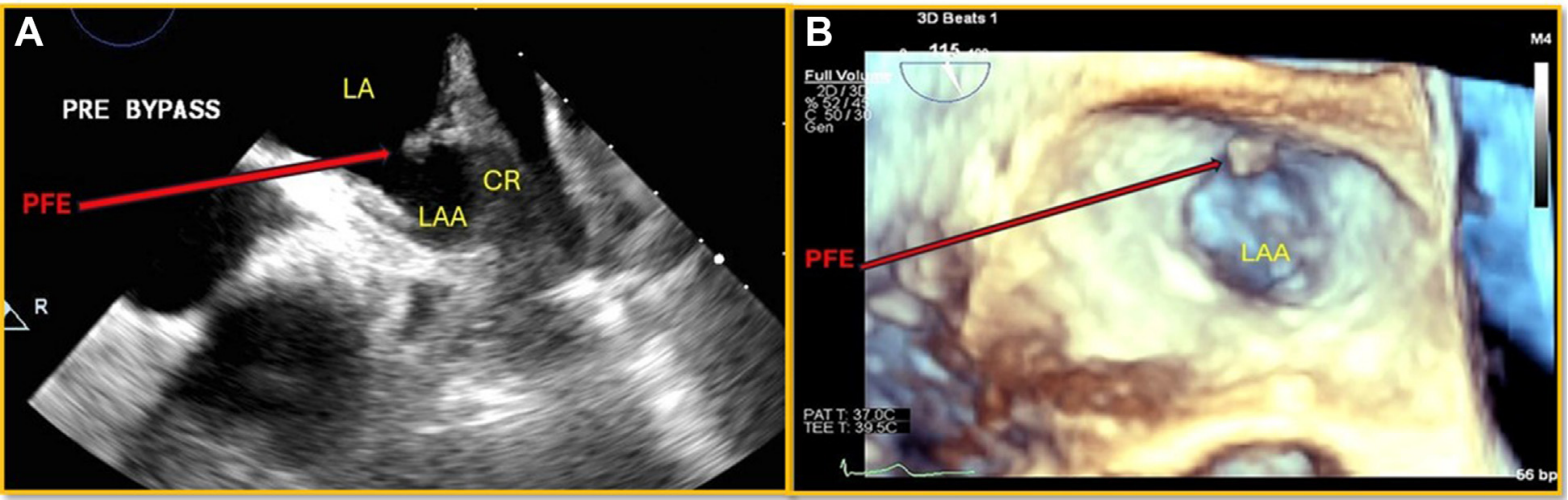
Transesophageal Echo (A) Intraoperative 2-D TEE: globular echodensity attached to the PVR. (B) 3-D TEE, en-face view of LAA orifice: mobile mass attached to the PVR. CR = coumadin ridge; PVR = pulmonary vein ridge; other abbreviations as in Figure 1.
